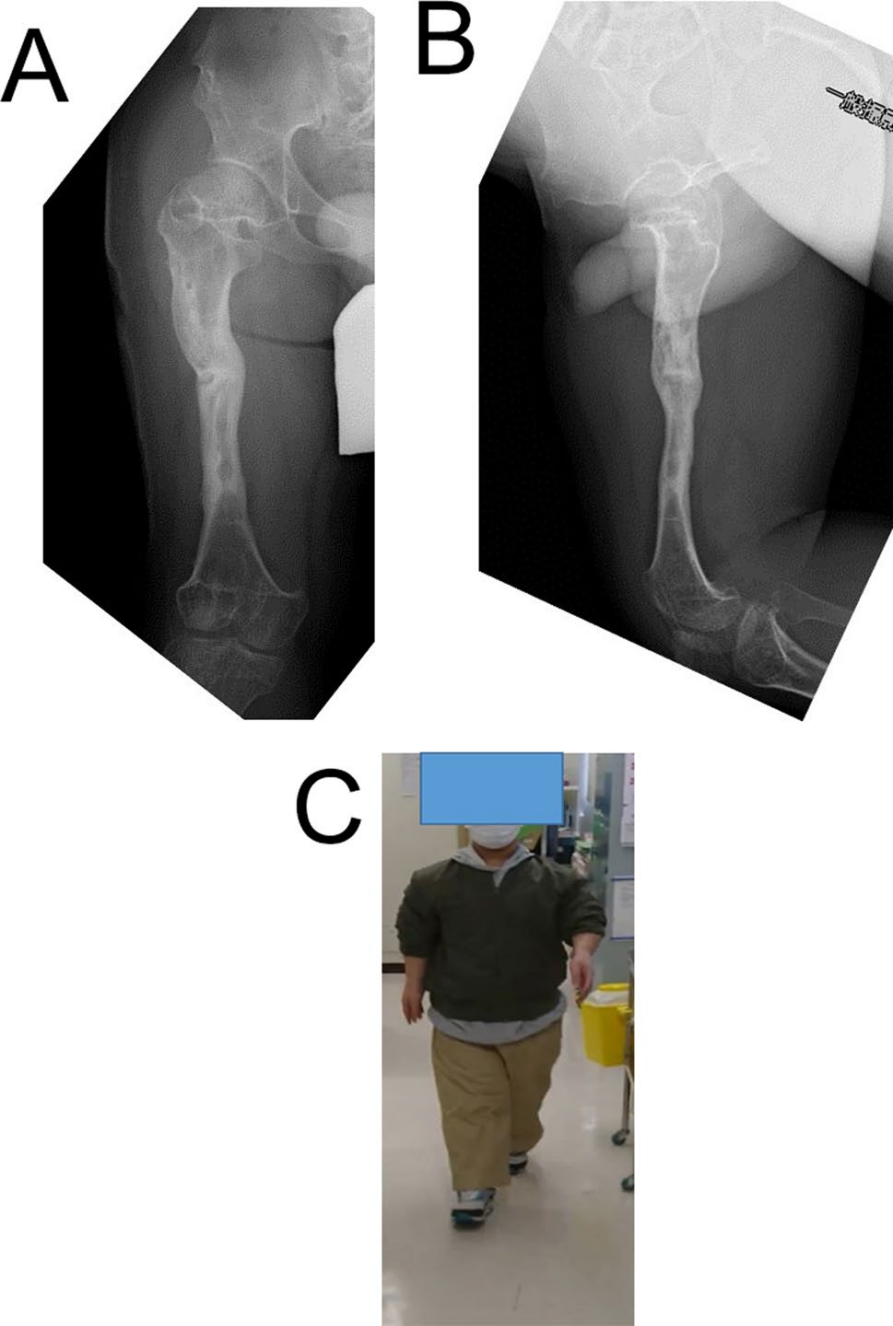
A, B: X-ray images taken at postoperative 7-year follow-up. C: Clinical photograph at postoperative 7-year follow-up
